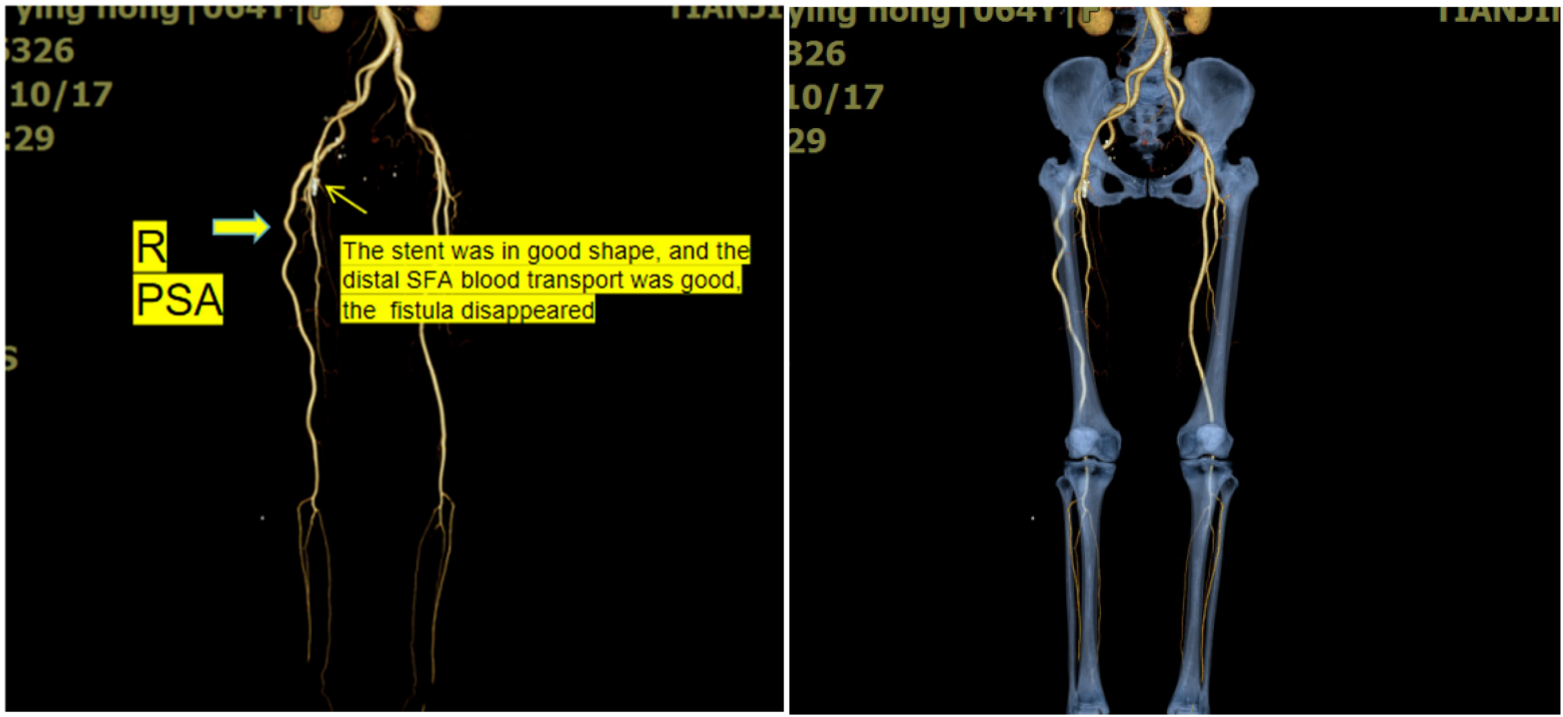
This series of pictures shows the CT imaging findings at 6-month follow-up.
